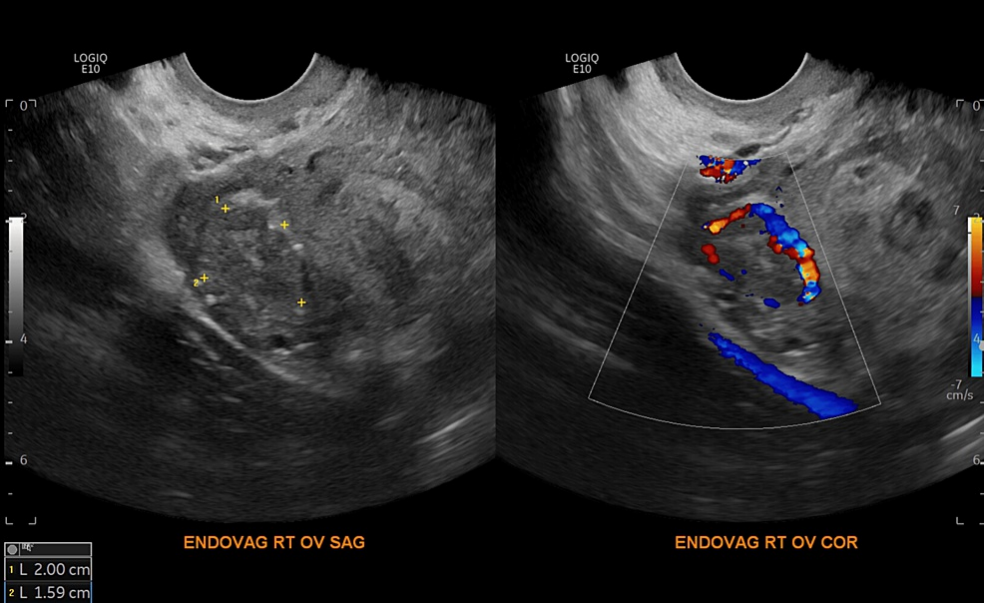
Transvaginal ultrasound of the right ovary in sagittal and coronal sections demonstrating a complex lesion with a “ring of fire” appearance using color Doppler.
